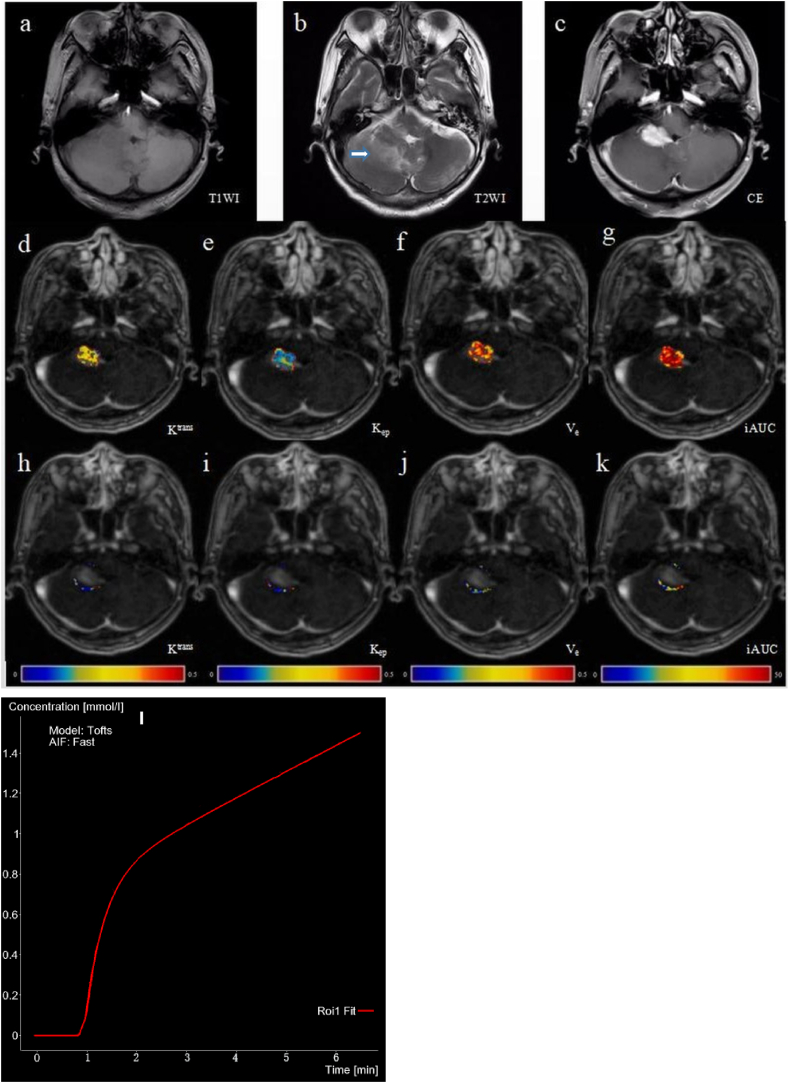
A 59-year-old male with primary central nervous system lymphoma (diffuse large B-cell lymphoma) in the right cerebellum. (a) T1-weighted image showing a slightly low signal. (b) T2-weighted image with a slightly high signal accompanied by peripheral moderate edema. (c) Enhanced scan displaying obvious enhancement. Pseudo-color maps of pharmacokinetic parameters in the enhanced tumor foci: (d) K^trans^ value of 0.232/min and its Ln value was −1.461; (e) K_ep_ value of 0.346/min and its Ln value was −1.061; (f) V_e_ value of 0.690 and its Ln value was −0.371; (g) iAUC value of 47.748 and its Ln value was 3.866. Pseudo-color maps of pharmacokinetic parameters in the peritumoral parenchyma: (h) K^trans^ value of 0.078/min and its Ln value was −2.551; (i) K_ep_ value of 0.185/min and its Ln value was −1.687; (j) V_e_ value of 0.052 and its Ln value was −2.957; (k) iAUC value of 4.964 and its Ln value was 1.602. (l) Fit graph of the enhancing tumor parenchyma based on the fast arterial input function (AIF) of the Tofts model. (For interpretation of the references to color in this figure legend, the reader is referred to the Web version of this article.)
